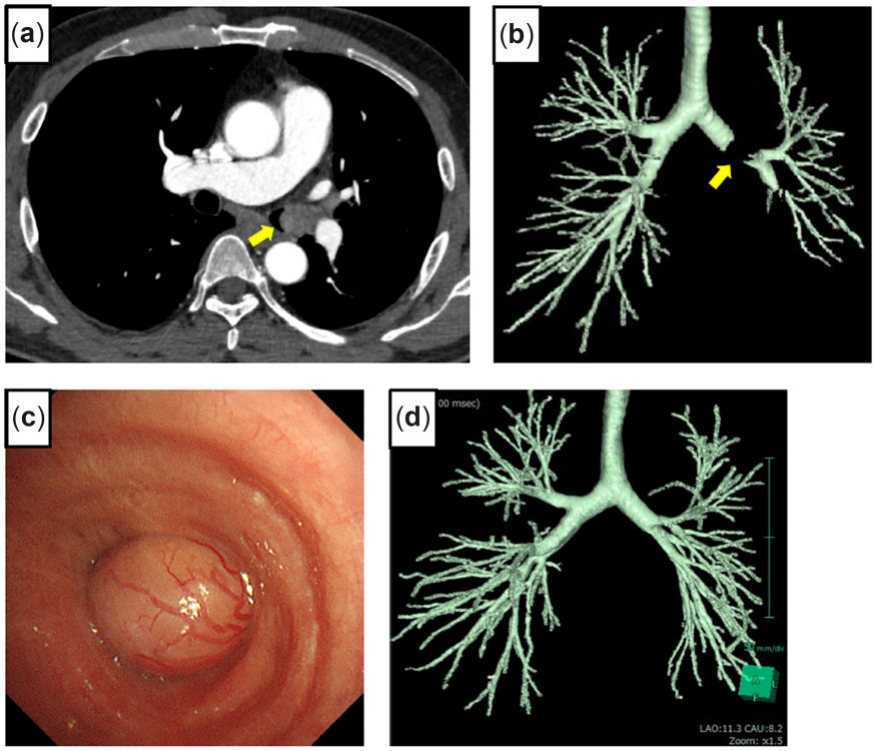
Chest computed tomography shows a tumour occupying the lower left main bronchus (arrow points to the tumour) (a). A 3-dimensional computed tomography scan shows almost total obstruction by the tumour (arrow points to the obstruction) (b). Bronchoscopy shows a tumour obstructing the lower left main bronchus (c). Postoperative 3-dimensional computed tomography shows airway lumen patency (d).
